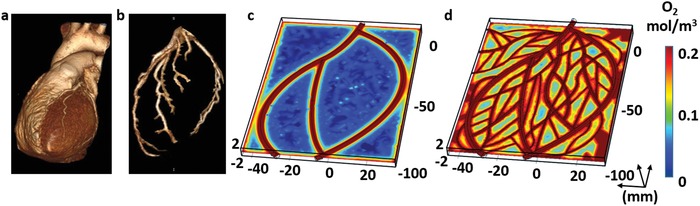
Imaging of the heart and patch modeling. CT image of a) a human heart and b) left ventricle coronary arteries. c) A model of oxygen concentration profile in an engineered patch. d) Replanning of the model showed better oxygen diffusion, sufficient to support cell viability.
